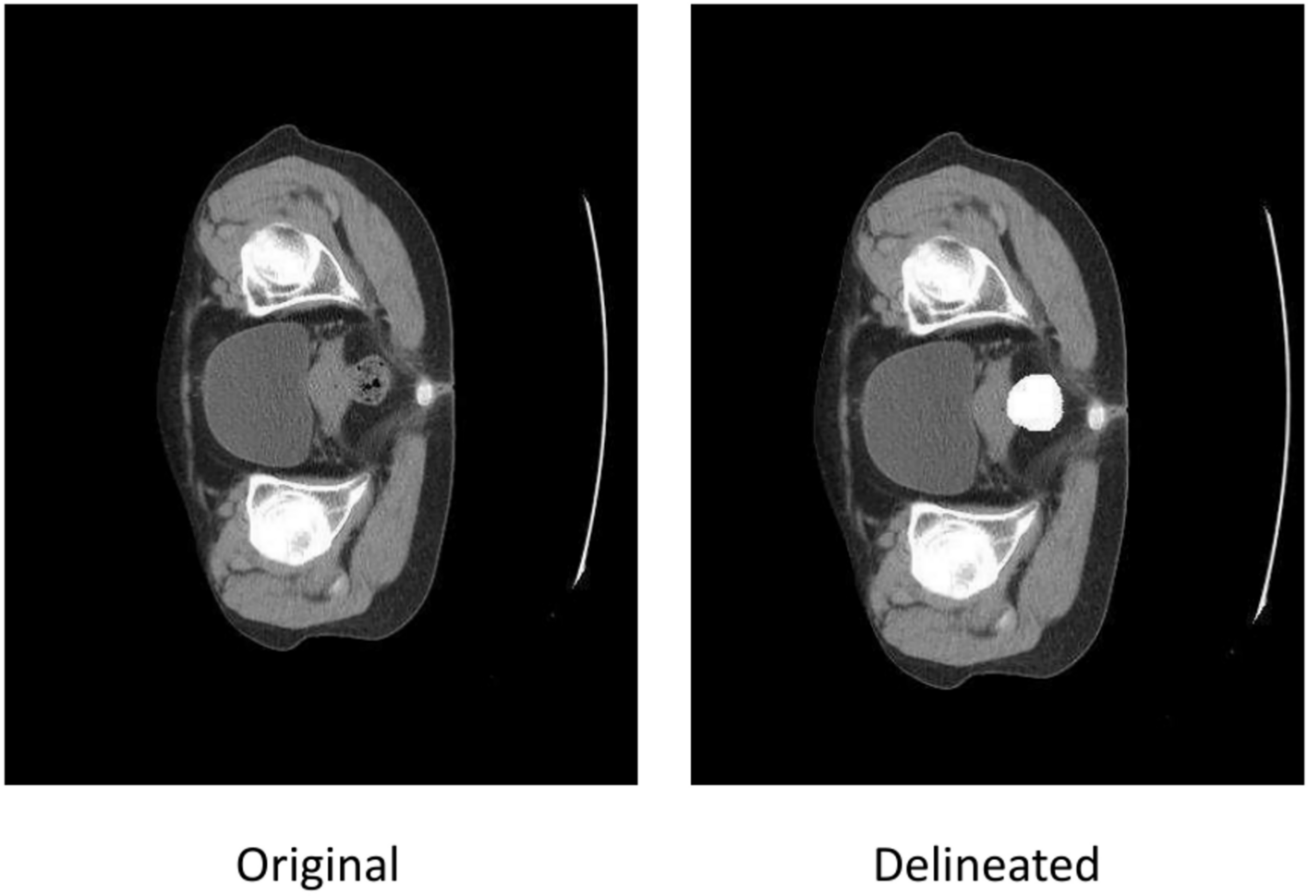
Overview of preprocessed CT data slices: the left image shows the patient’s CT data, while the right image highlights the rectal region delineated for that patient.
